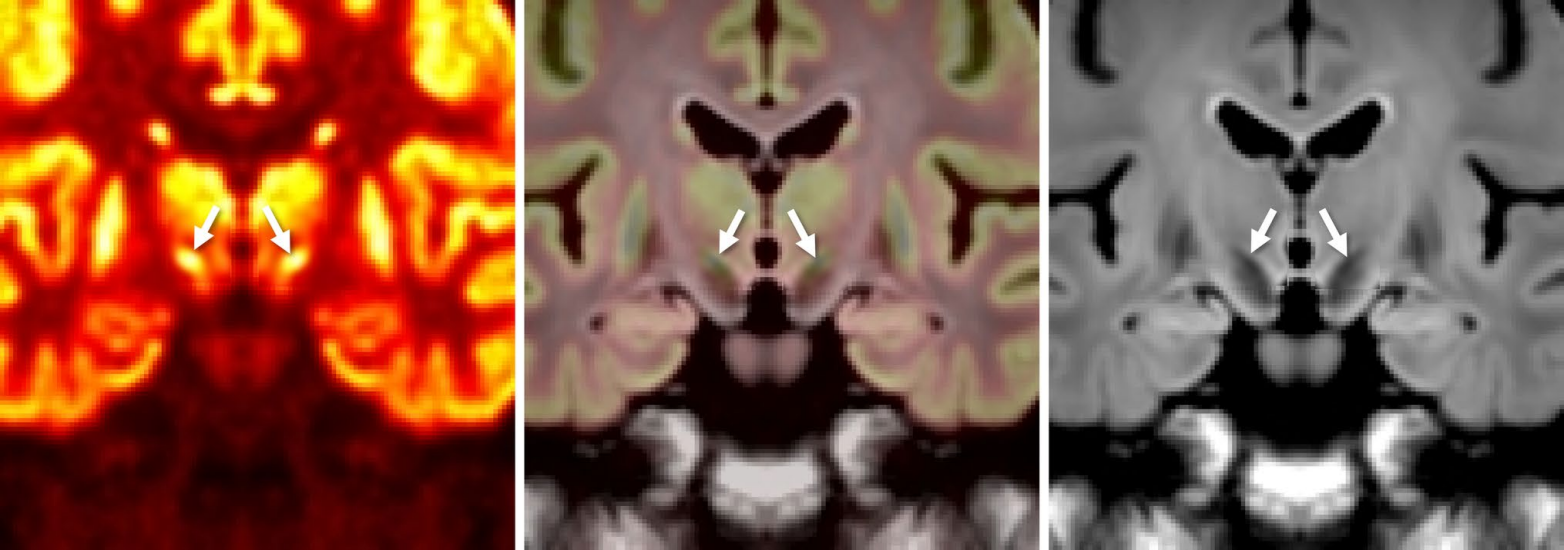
^18^F-FDG accumulation in subthalamic nuclei. Coronal average ^18^F-FDG PET image with reconstruction of OSEM iteration 64 + TOF + PSF (left, arrows) demonstrates avid FDG uptake corresponding to subthalamic nuclei (^18^F-FDG PET/3D-FLAIR fusion image, middle, arrows), which are well-recognized on coronal 3D-FLAIR images (right, arrows)
